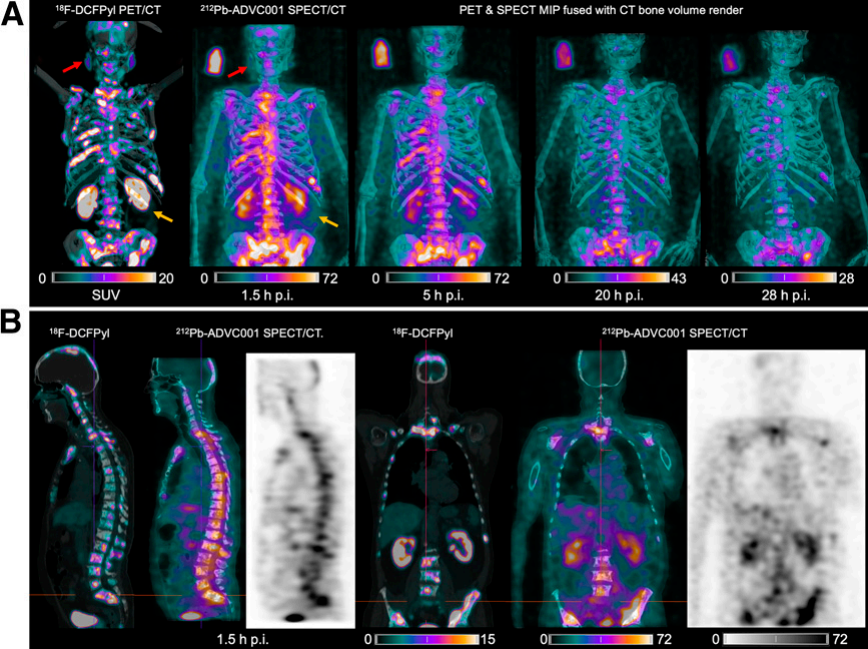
(A) ^18^F-DCFPyl PET/CT and ^212^Pb SPECT/CT images showing concordant tumor biodistribution with low salivary gland uptake (red arrow) and rapid kidney clearance of 60 MBq of ^212^Pb-ADVC001 (structure of ^212^Pb-ADVC001 available as supplemental material at http://jnm.snmjournals.org). A 3-MBq standard solution (100 mL) was included. (B) Sagittal and coronal images at 1.5 h after injection (p.i.). MIP = maximum-intensity projection.
